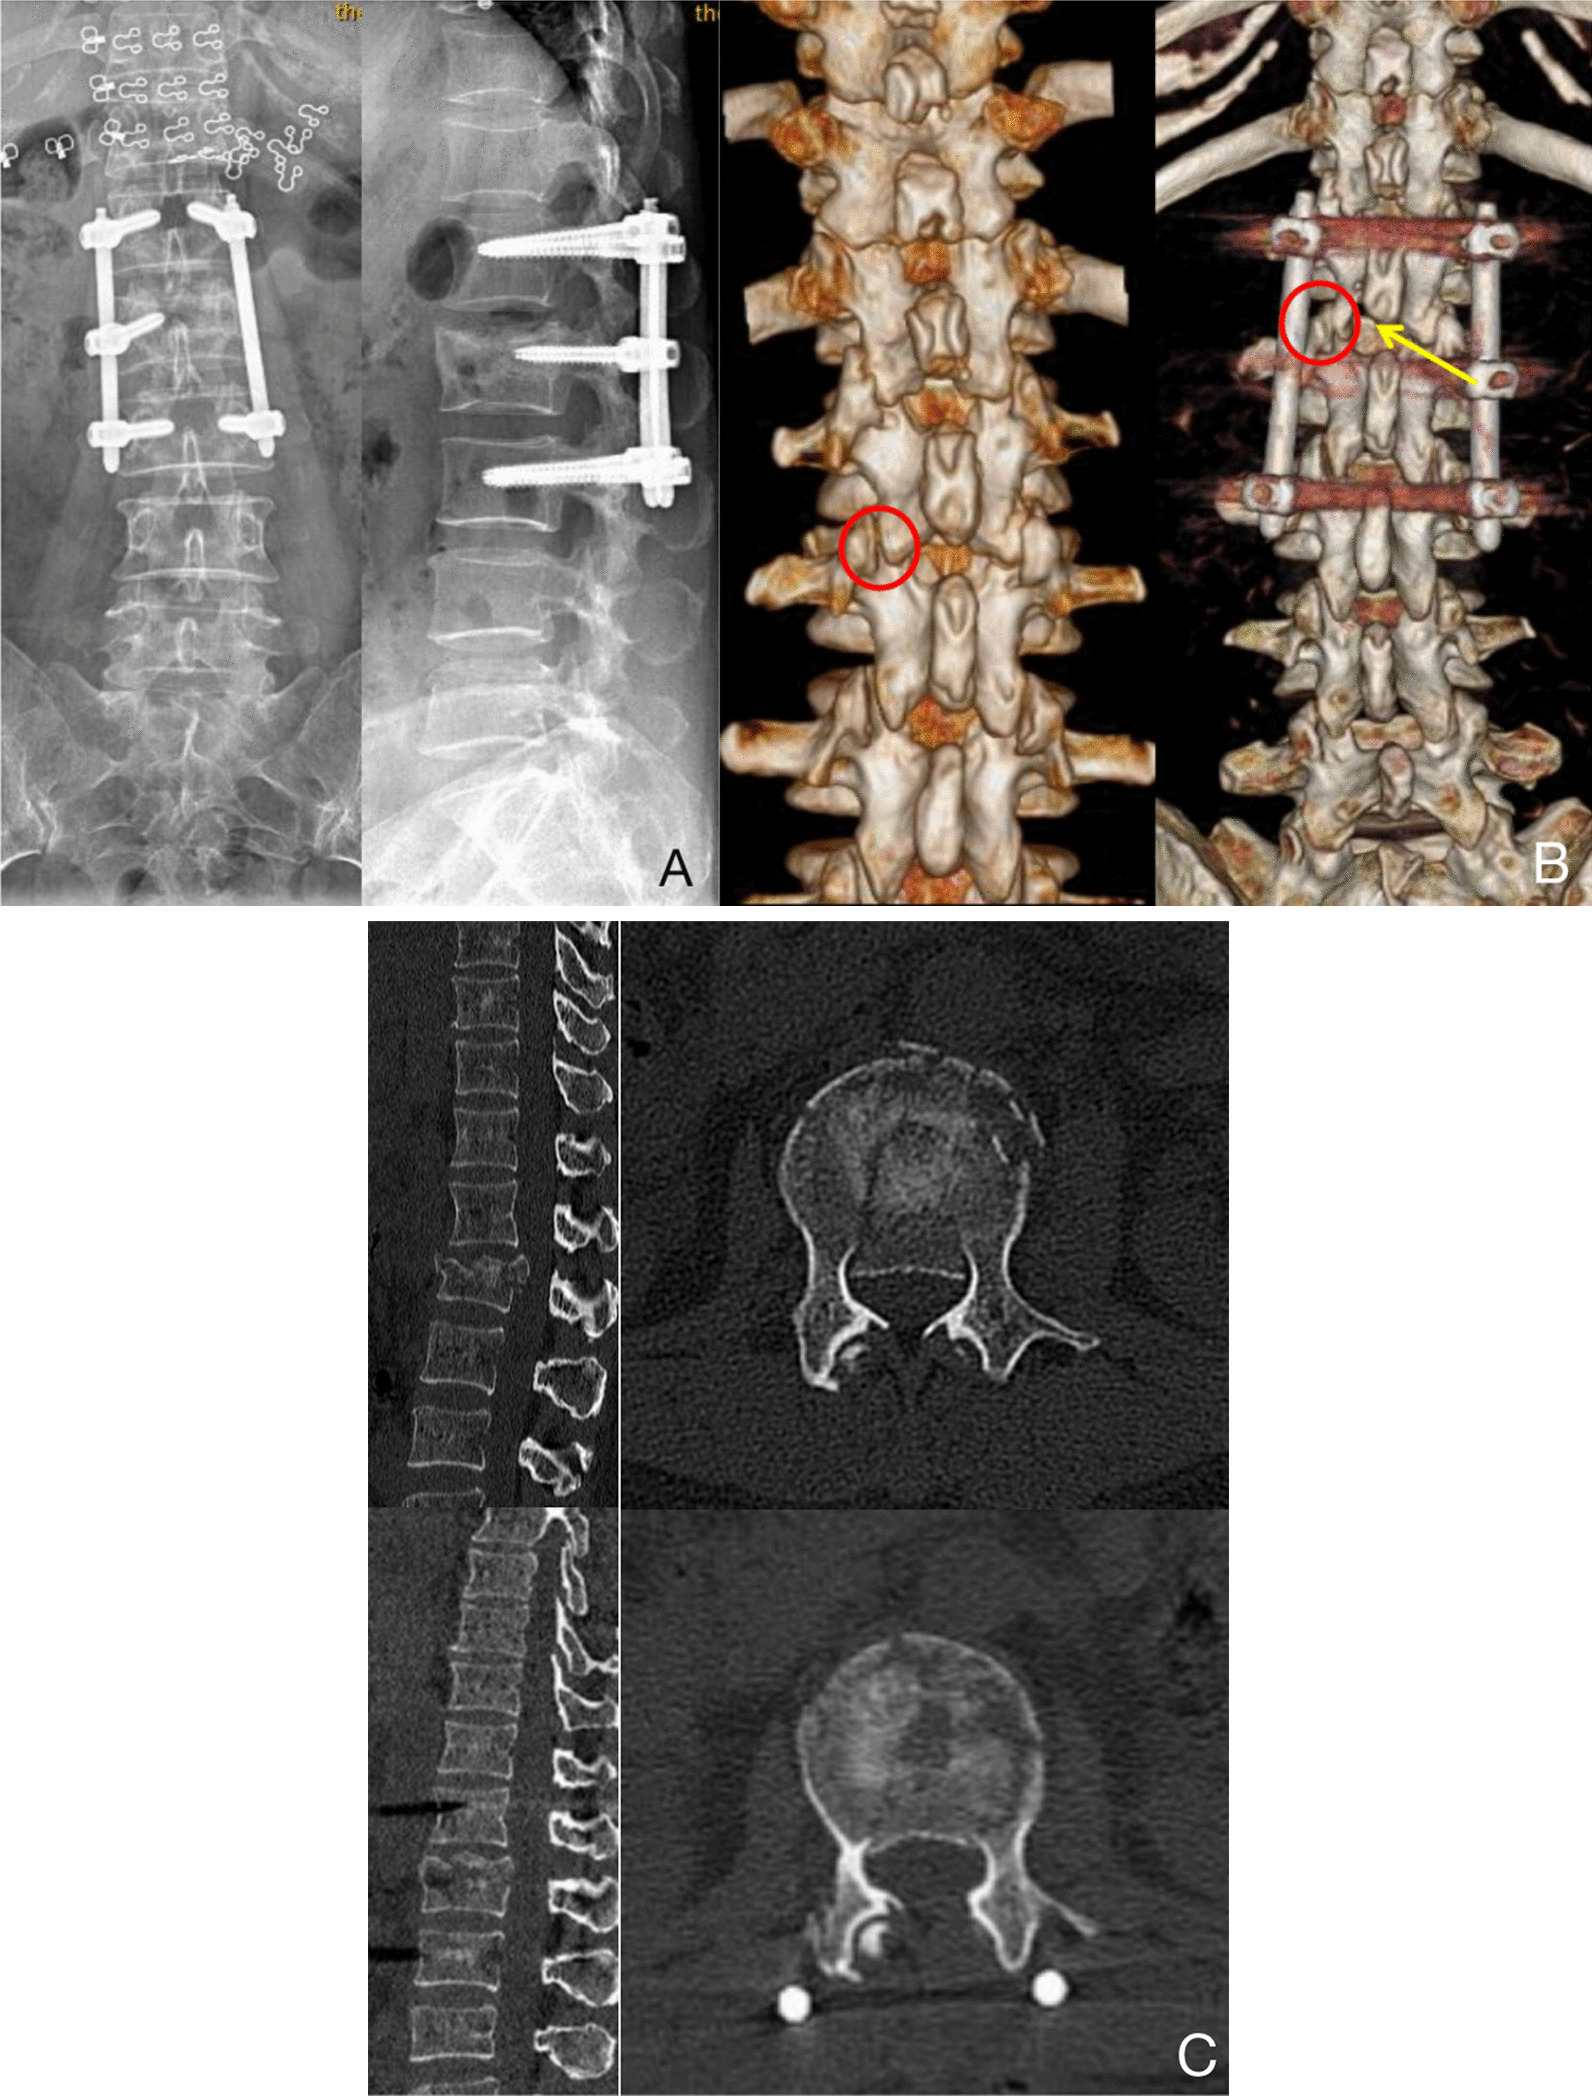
A Postoperative radiograph. The fractured vertebra is reduced, and the position of the screw is good. B Postoperative 3D images show good lamina fenestration (y*ellow arrow*) and facet joint protection (*red circle*). C Lumbar computed tomography scan sagittal and axial views demonstrate the protruding bone is successfully reduced
